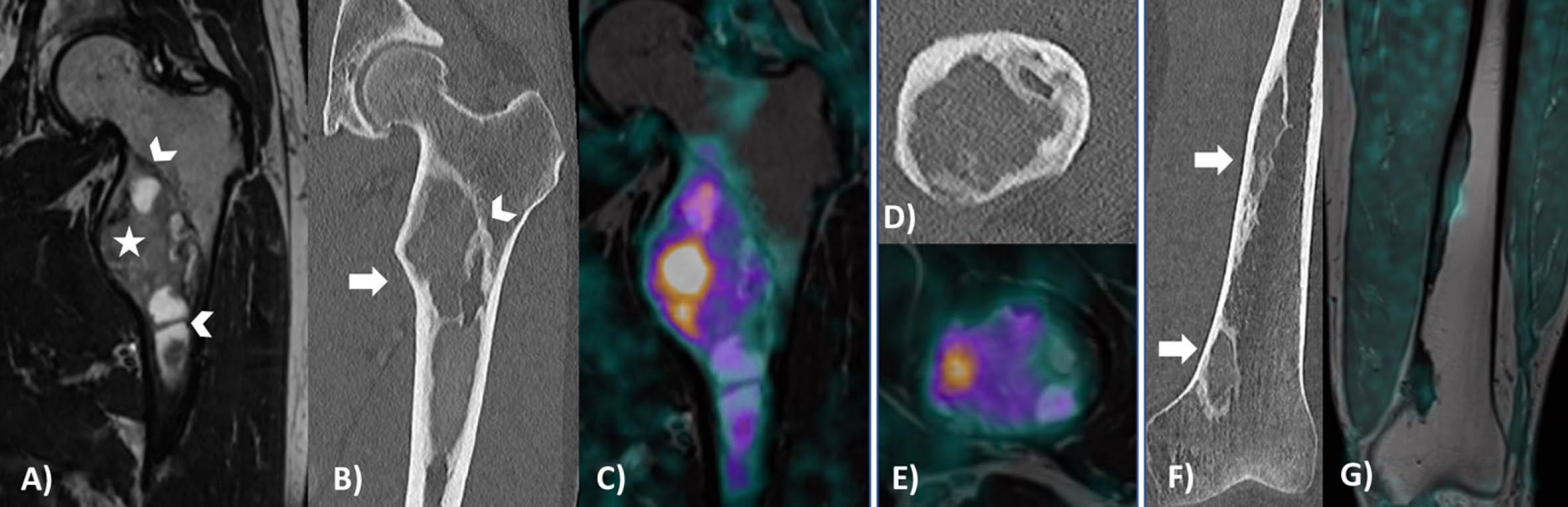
Cross-sectional imaging of left femoral bone lesions. T2-weighted, coronal MR (A), CT (B), and PET/MR (C) images showing a large lesion of the lesser trochanter and proximal diaphysis of the left femur, characterized by increased FDG metabolism corresponding to the viable portion of the tumour. Axial CT suggests cortical interruption of the posterior border of the lesser trochanter (D), corresponding to metabolically inactive tissue, as shown by PET/MRI (E). Coronal CT (F) showing two additional osteolytic lesions in the distal diaphysis and in the distal metaphysis of the left femur, respectively, with well-defined sclerotic borders and no periosteal reaction. T1-weighted PET/MRI fusion sequence (G) shows no significant FDG uptake corresponding to these additional lesions. FDG images were adjusted to the same intensity colour scale
